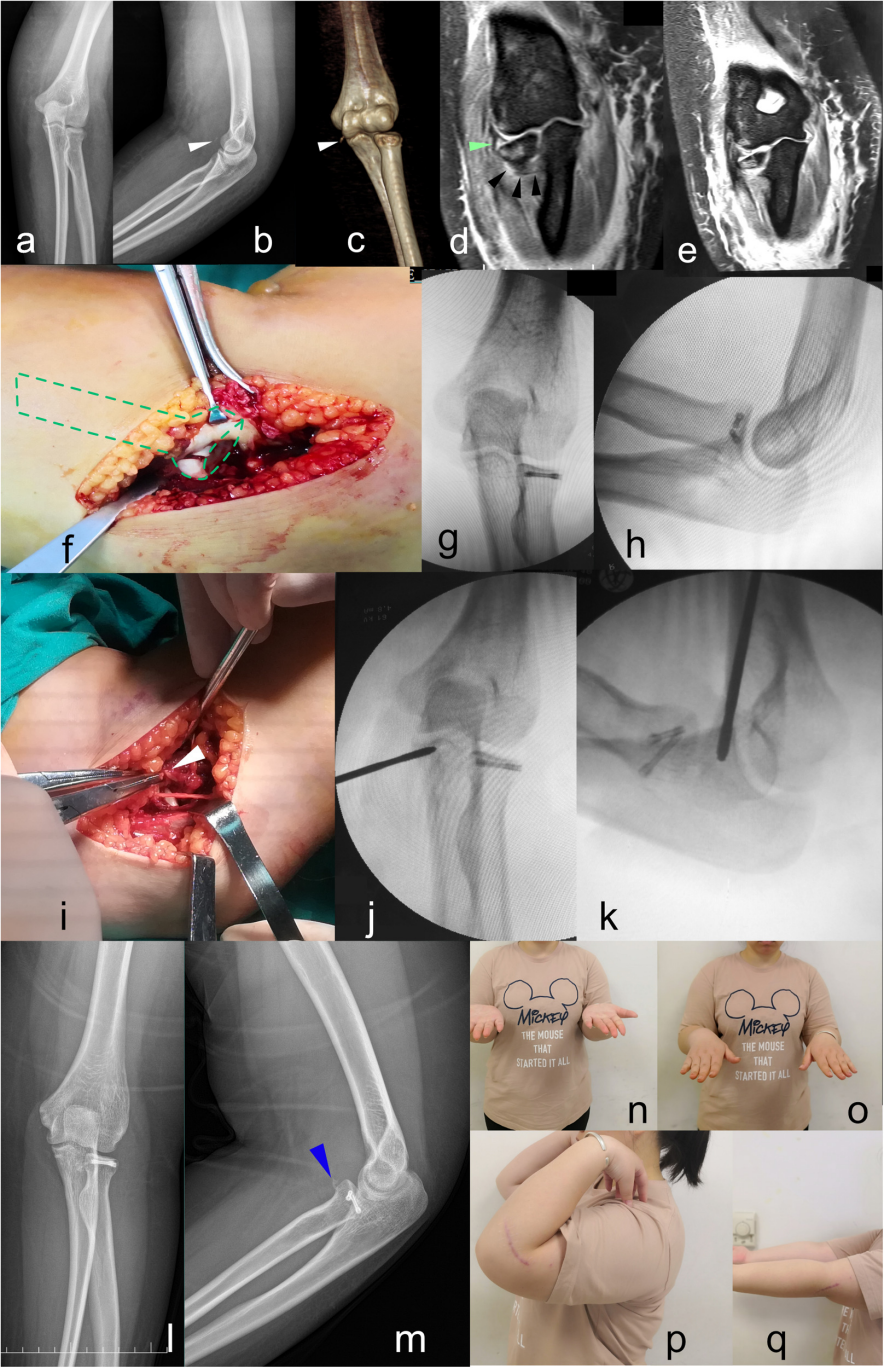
A 23-year-old woman (case 2) with valgus-type TTI. Dislocation history was reported by the patient. Preoperative radiographs (a–e) showed lateral angulation of the radial head, medial avulsed fragment (white triangle), intact LUCL (black triangle), and intact radial collateral ligament (green triangle). Bone contusion was found less extensive in the medial half of the elbow joint (e). Joint instability only recurred under valgus stress. Intraoperatively, the radial head was fixed by two screws (f–h). The anterior bundle of the MCL had avulsed and was repaired by a suture anchor (i–k). At the 1-year follow-up, the MCL avulsion non-union (blue triangle) did not affect joint function (n–q).
